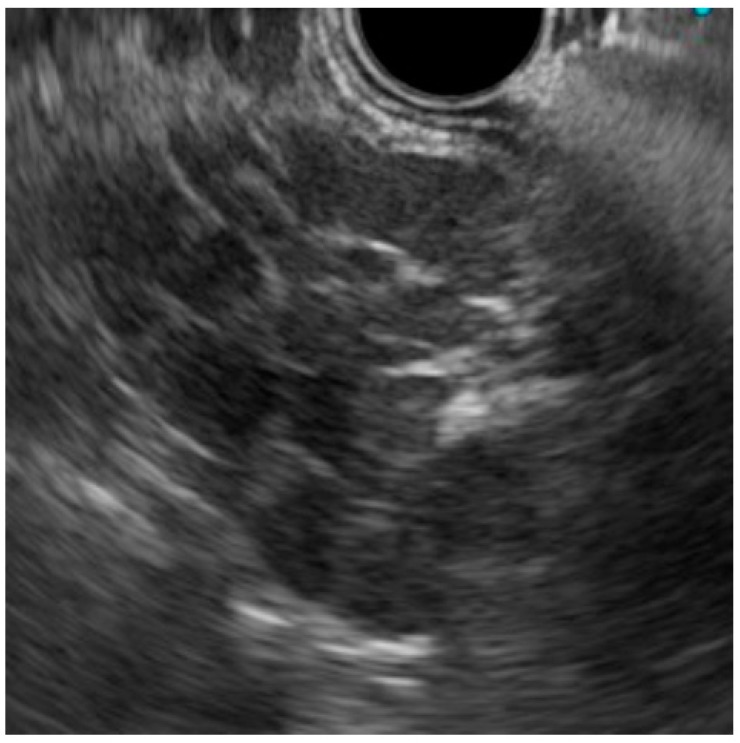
Endoscopic ultrasonography (EUS) demonstrating a mass lesion composed of multiple aggregated low-echoic nodules.
